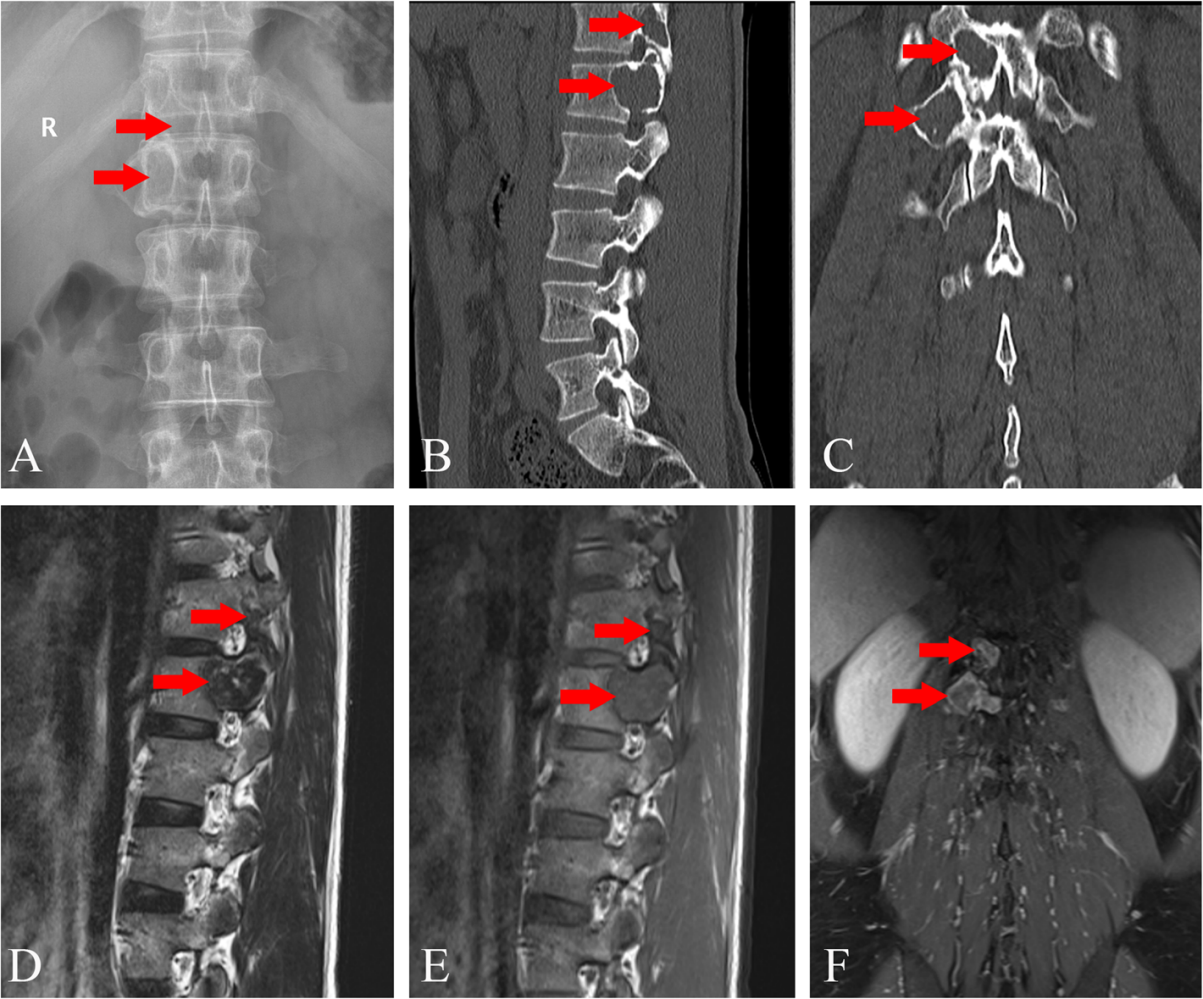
a. X-ray reveals lesions at T12 and L1 vertebrae; b. Sagittal and c. coronal CT scan reveals osteolytic lesions; The lesions are d. T1-weighted and e. T2-weighted iso- and hypo-intensities, with f. ring-enhancement of the peripheral area on MRI
